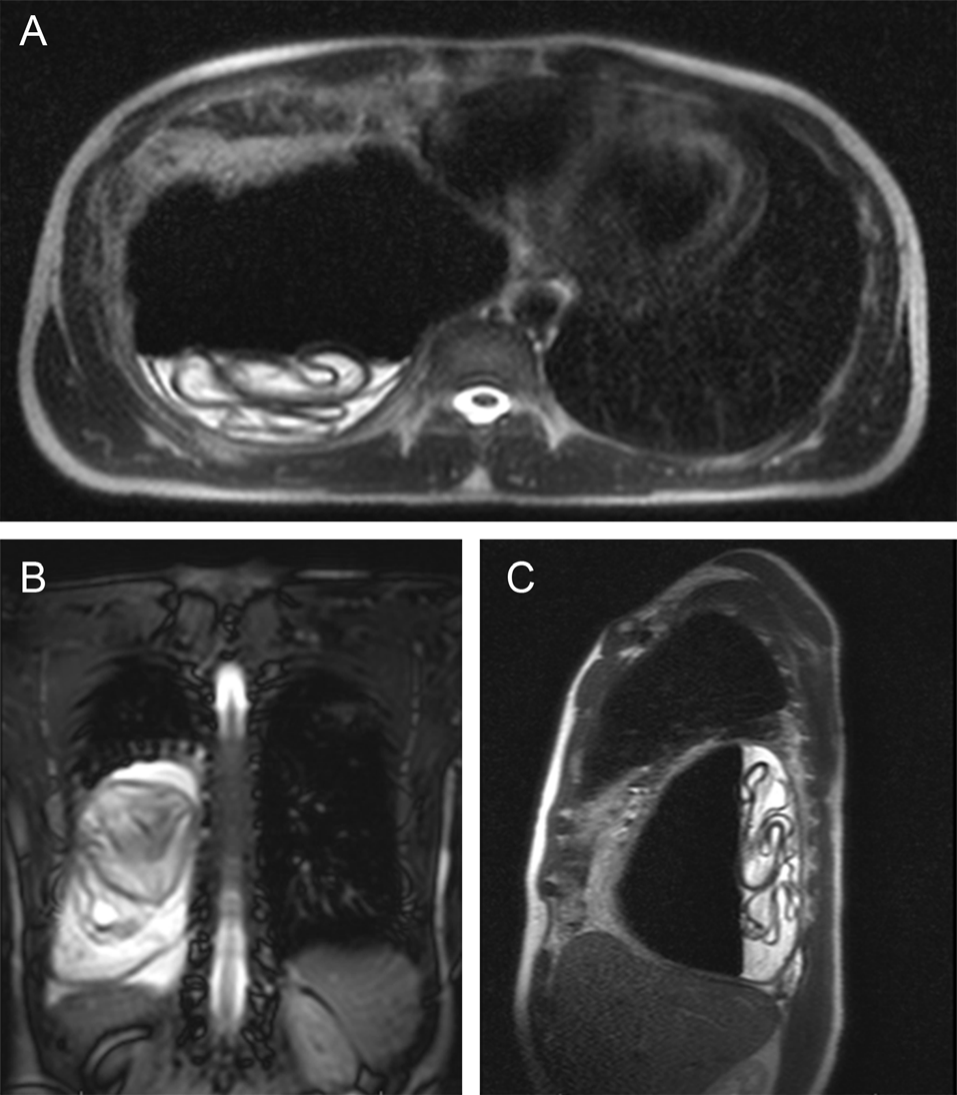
Axial (A), coronal (B), and sagittal (C) T2-weighted magnetic resonance image shows rupture of giant hydatid cyst in the right lung.
